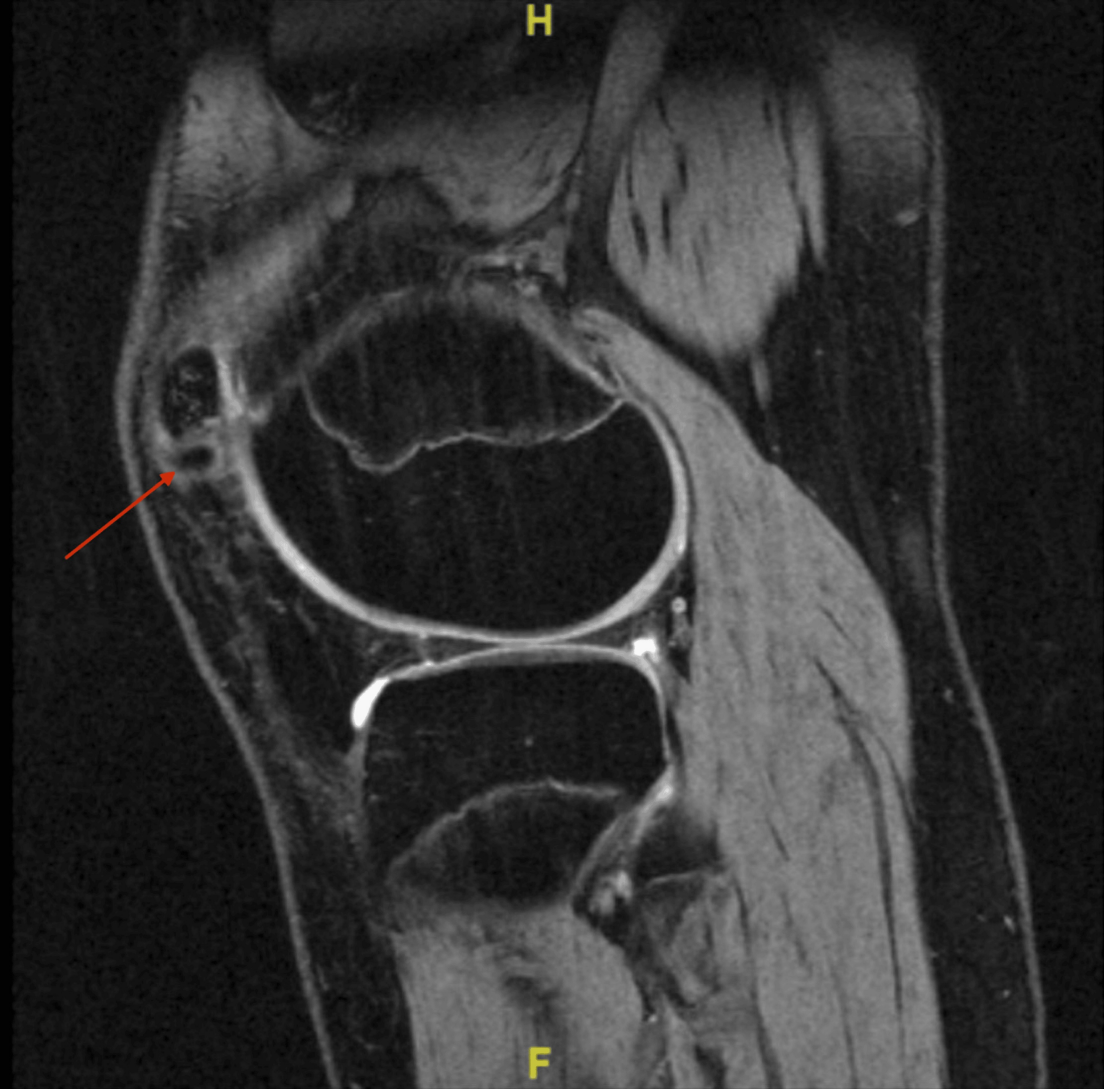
MRI without contrast of L knee showing superolateral portion of bipartite patella
